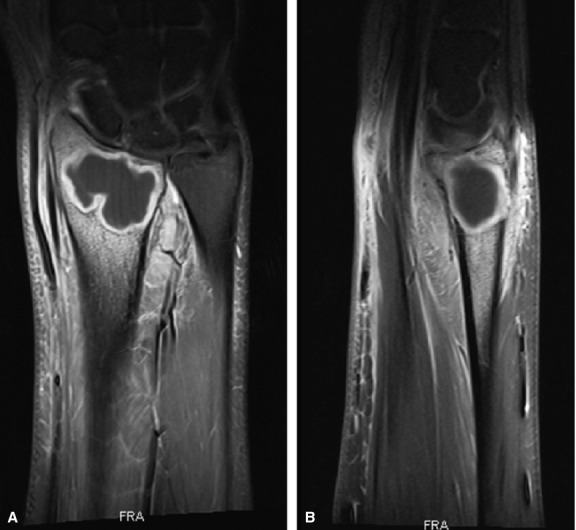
Pre-operative MRI of the lower arm (case 2) showing cystic formations at the distal radius consistent with a giant cell carcinoma. (A) Coronar reconstruction. (B) Sagittal reconstruction.
